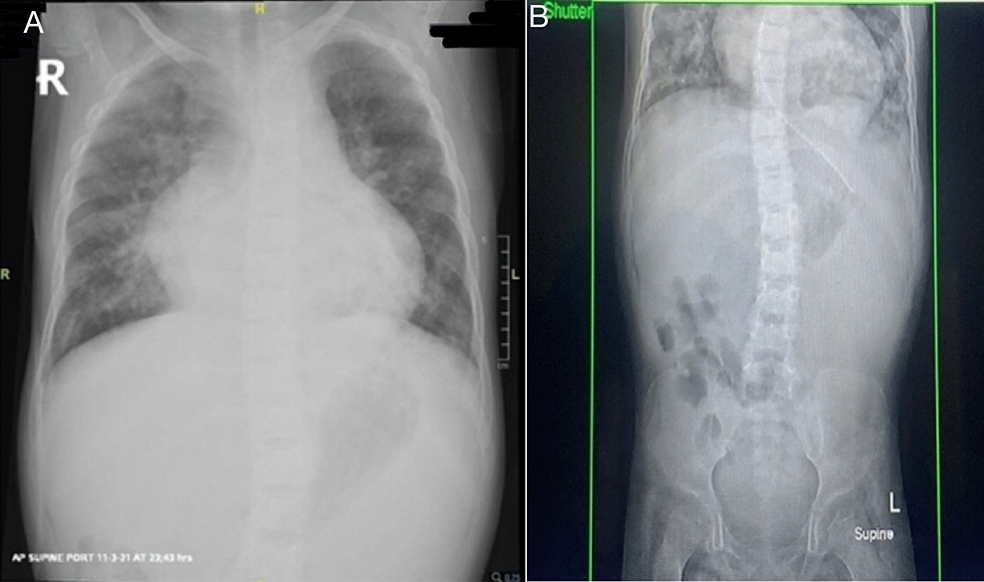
(A) Chest X-ray showing cardiomegaly and (B) abdominal X-ray showing scoliosis.
